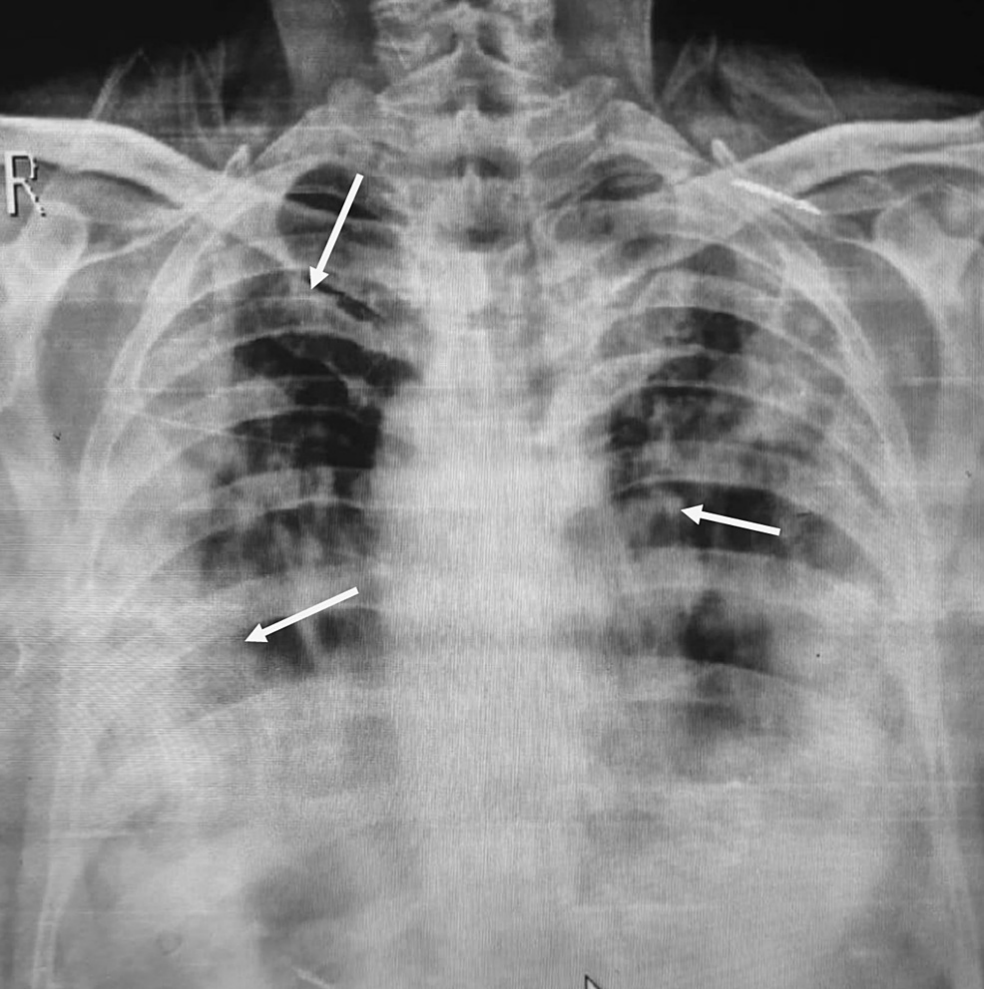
Chest x-ray showing diffuse infiltrates in both lung fields.
